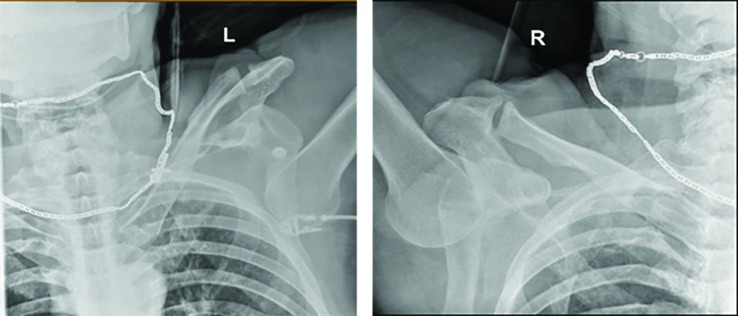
Bilateral shoulder radiography taken on admission which demonstrates bilateral luxatio erecta
